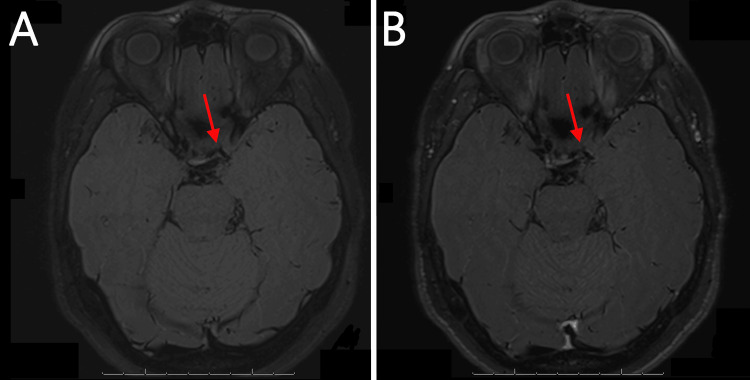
Magnetic resonance imaging (MRI) with vessel wall imaging (A) Precontrast and (B) postcontrast delays alternating with nutation for tailored excitation (DANTE) MRI sequences demonstrated ≥60% stenosis (WASID study criteria) of the left distal ICA (arrows) with associated hyperintensity of the vessel wall but no gadolinium enhancement. WASID: Warfarin–Aspirin Symptomatic Intracranial Disease; ICA: Internal carotid artery.
